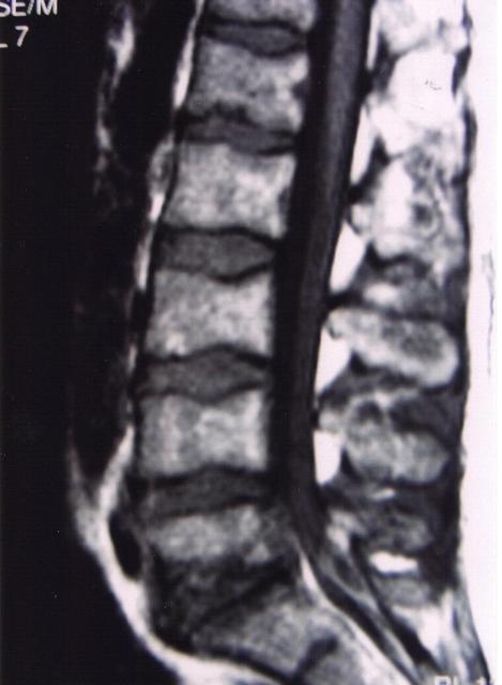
A sagittal pre-contrast T1-weighted spin-echo (SE) image shows a fluid collection in the posterior epidural space with low signal intensity, that is anteriorly compressing the dorsal sac and decreased signal intensity of the L5 and S1 vertebral bodies.
